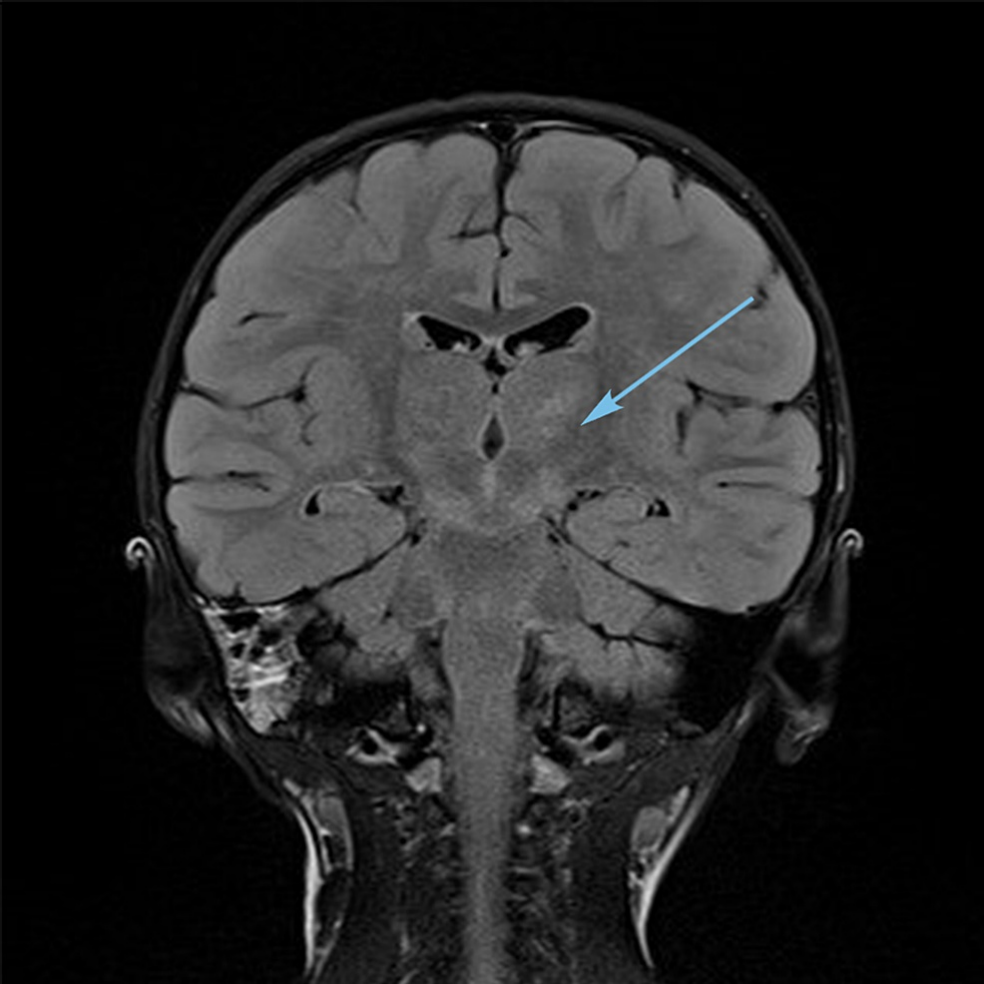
MRI brain T2 coronal FLAIR image showing thalamic and subthalamic hyperintensities (arrow). FLAIR, fluid-attenuated inversion recovery.
